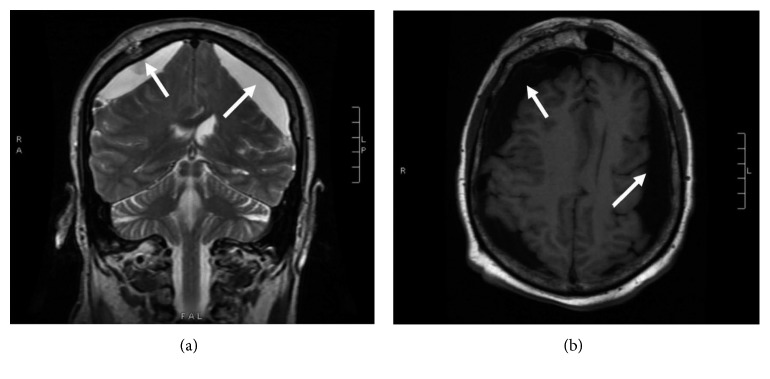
(a) T2-weighted coronal sequence from an MRI of the brain. (b) An axial T1 flair image.
